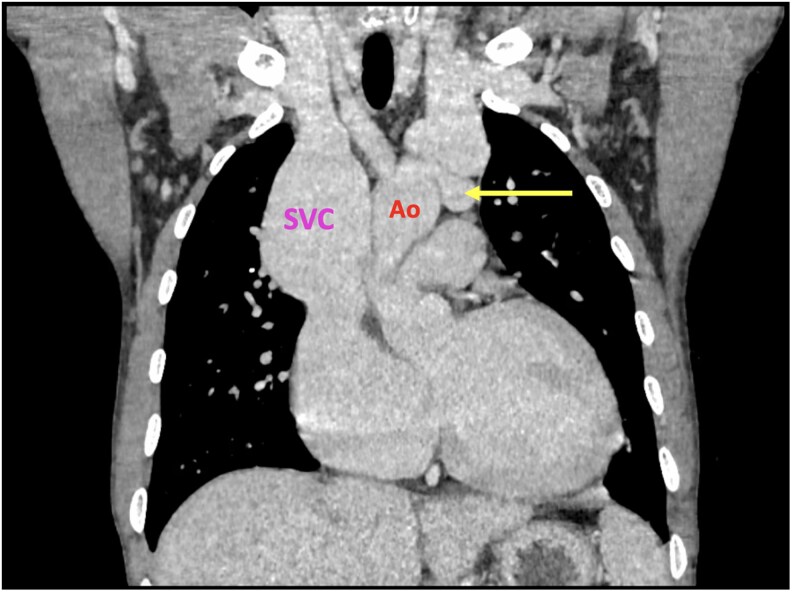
Computerized tomography (CT) coronary view. The yellow arrow indicates the arteriovenous fistula. SVC, superior vena cava; Ao, aorta.
